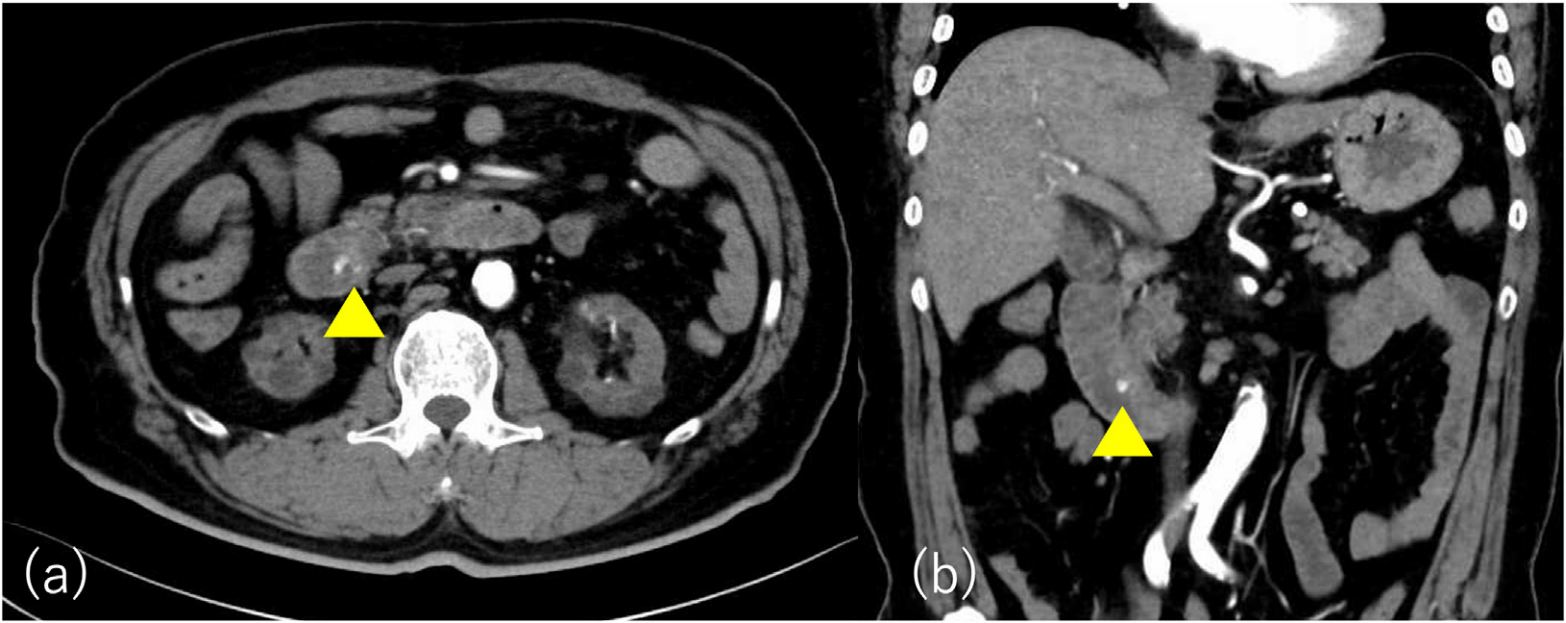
Abdominal contrast-enhanced computed tomography after hematemesis the day after the procedure. Abdominal contrast-enhanced computed tomography showing contrast leakage (yellow arrowheads) into the descending duodenal limb in axial (A) and coronal (B) views.
